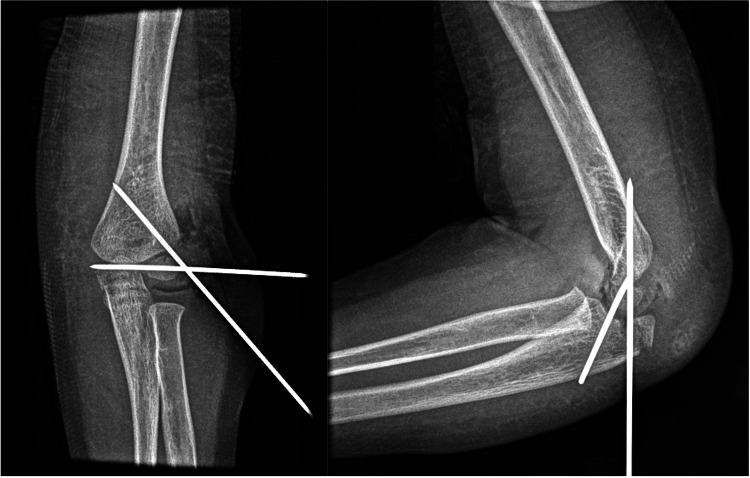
Immediate postoperative radiographs (A) Anteroposterior and (B) lateral radiographs of the elbow, revealing a successful reduction of the lateral condyle fracture and proper positioning of the Kirschner wires Importantly, the olecranon fracture sustained no displacement
